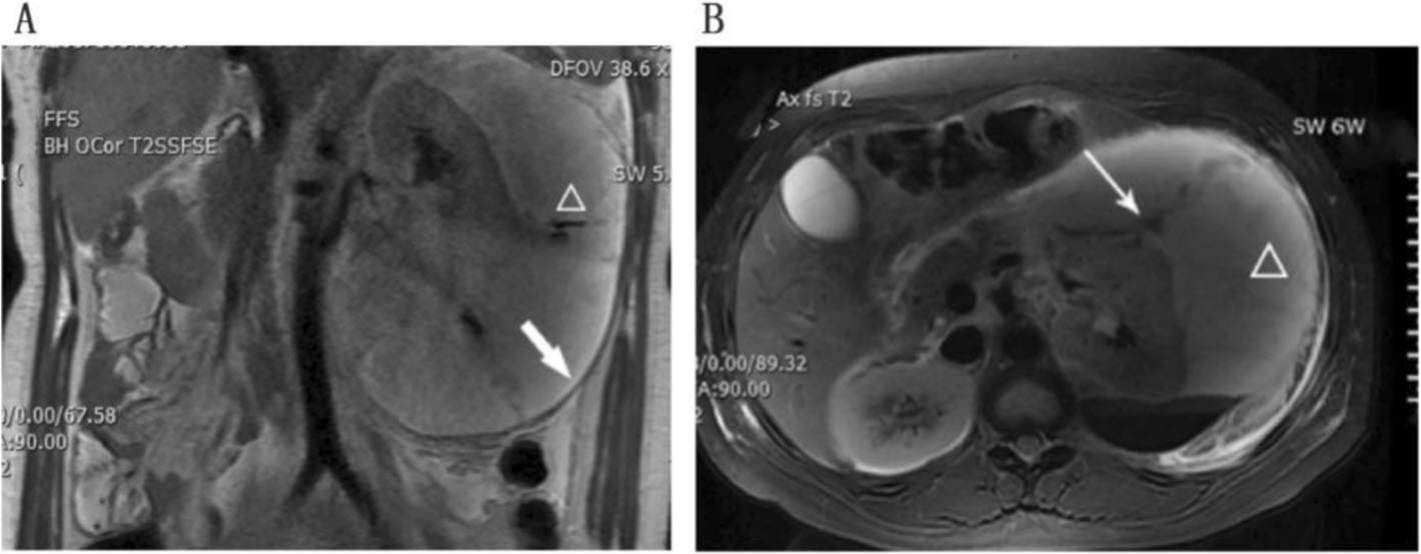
a Coronal slice of MRI on the first visit, the image showed the fluid(△) localized under the left renal capsule (→). b Axial image of MRI on the first visit showed the rupture of her left kidney (→) and the fluid extravasation around it (△)
